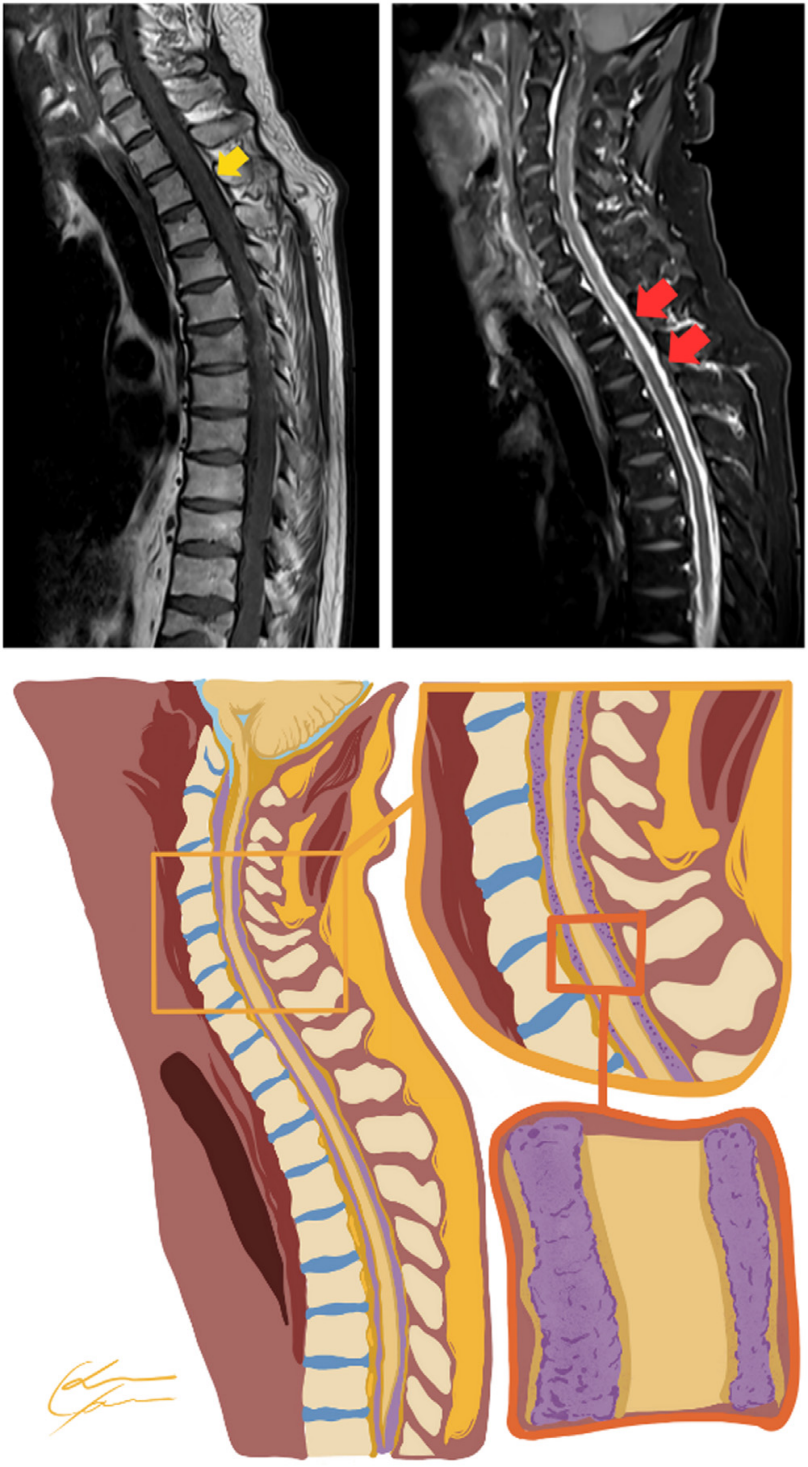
Sagittal T1 before (top left) and after contrast (top right). Sequences with diffuse and thick leptomeningeal enhancement involving the spinal cord. At the bottom, a graphic representation of the magnetic resonance imaging (MRI) findings with infiltration of the leptomeninges around the spinal cord.
